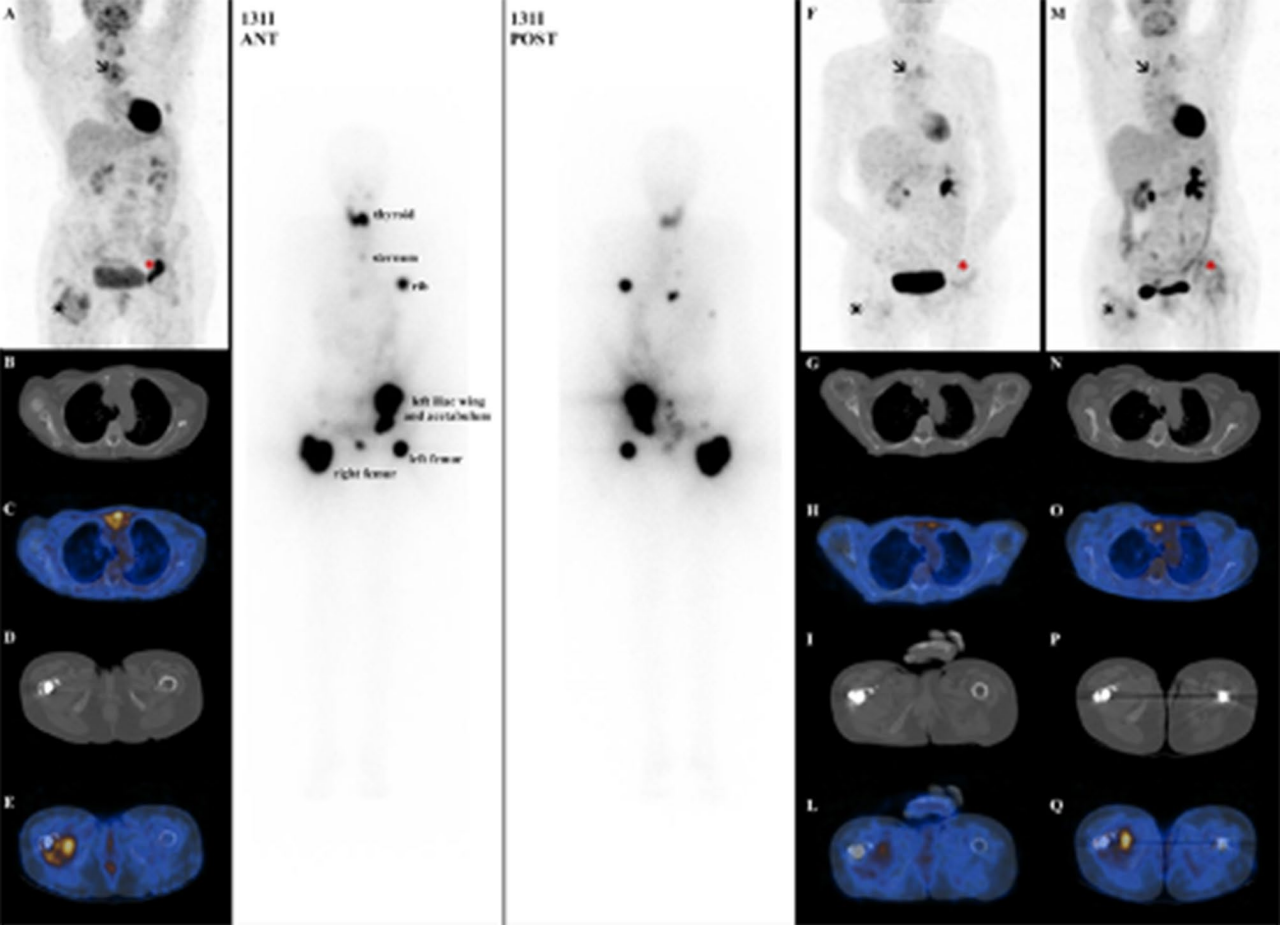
Case presentation of a 67-year-old female patient with metastatic PTC. (a–e) images show baseline 18F-FDG PET/CT scans. After the diagnosis, she underwent total thyroidectomy (pT3Nx) with a partial resection of the sternum metastasis, and RAI treatment (activity 5.5 GBq). The 131I whole-body scan (131I ANT and 131I POST) showed RAI uptake in all metastases detected at the baseline 18F-FDG PET/CT, with an heterogenous FDG-avidity (131I+/18F-FDG+). A second RAI treatment was not performed for persistent neutropenia; for this reason, the patient was candidate for locoregional therapy (RFA of the left iliac wing and left acetabulum) and she was followed-up with 18F-FDG PET/CT. The first follow-up 18F-FDG PET/CT scan (f–l) demonstrated a very good metabolic partial response of the left iliac wing and left acetabulum BM (red asterisk), with also a decreased tracer uptake at the right femur level (black asterisk). Despite that, 18F-FDG PET/CT scan performed after 12 months of follow-up (m–q) showed disease progression, with the appearance of new increased tracer uptake in all the previous sites of disease (sternum, left iliac wing and left acetabulum and right femur). *PTC* papillary thyroid cancer, *18F-FDG PET/CT* 18F-fluorodeoxyglucose positron emission tomography/computed tomography, *RAI* radioactive iodine, *RFA* radiofrequency ablation, *BM* bone metastases
